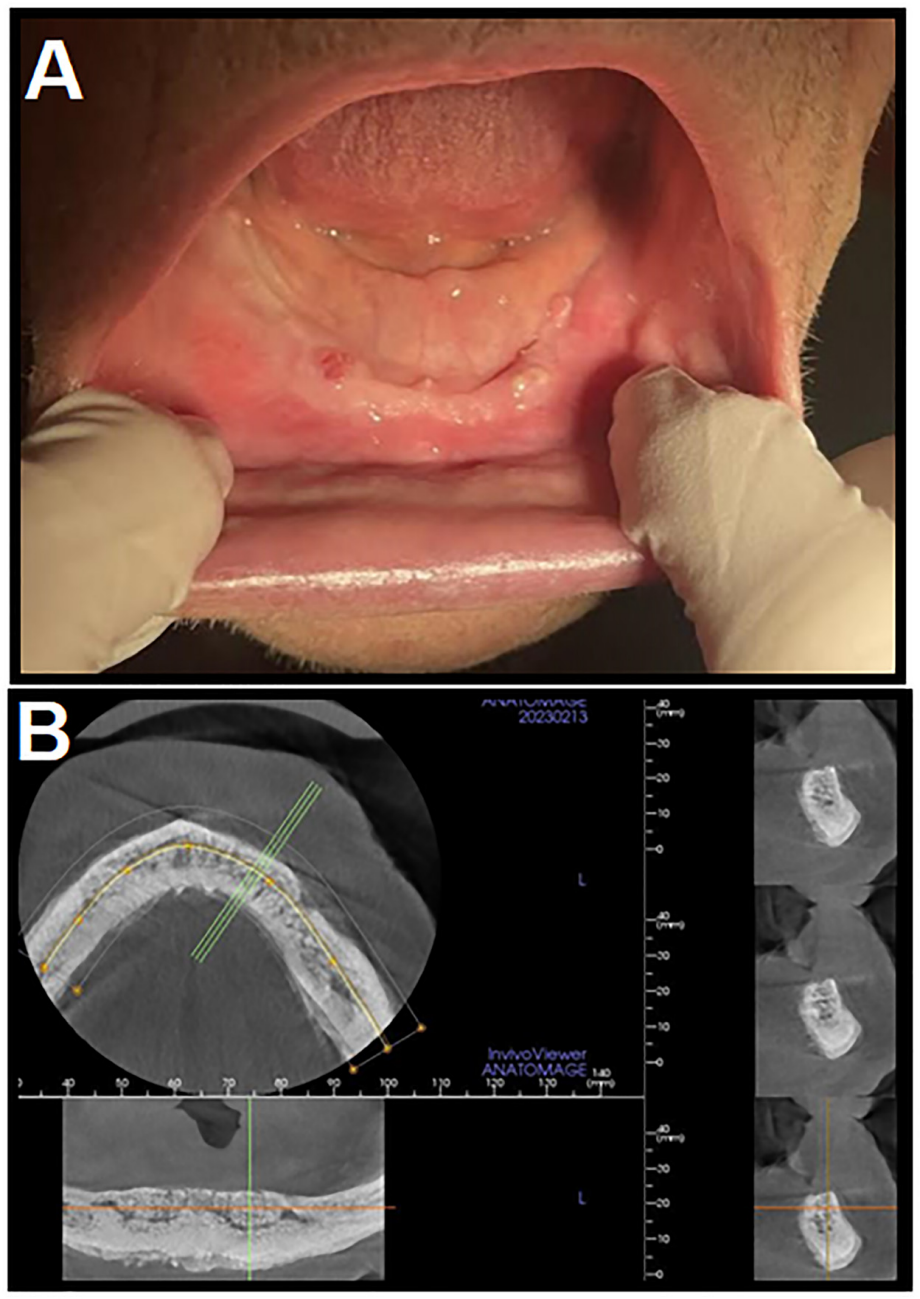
Clinical images corresponding to Case 3 A: Clinical presentation of the patient during surgery; B: Cone-beam CT (CBCT) image of patient before the surgical intervention
